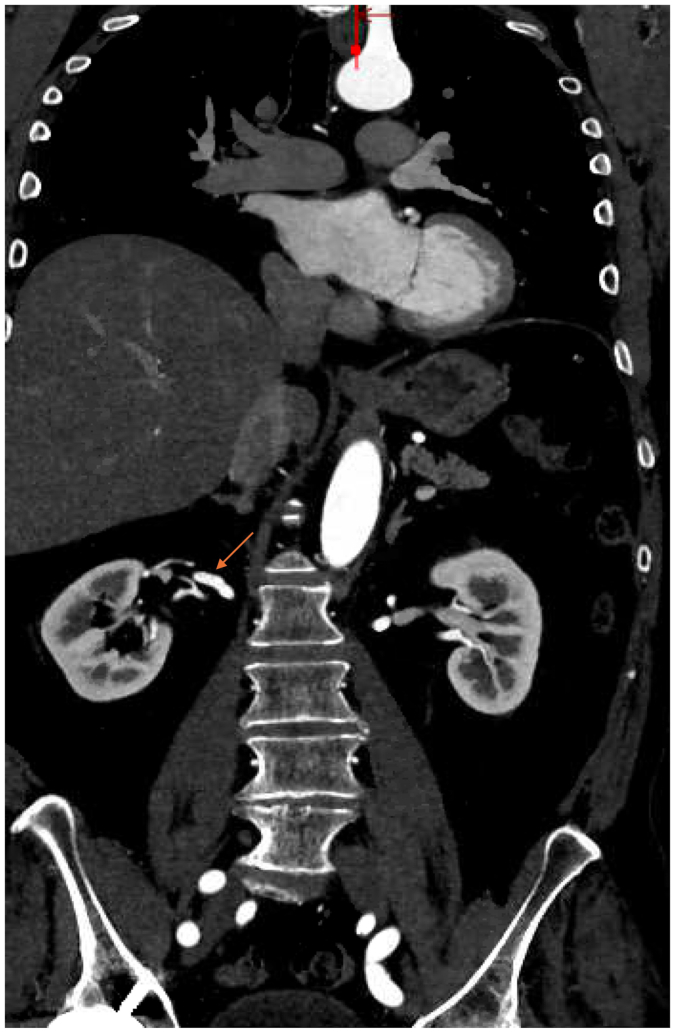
Computed tomography angiogram (CTA) sagittal view showing right renal artery beading appearance, *orange arrow*.
